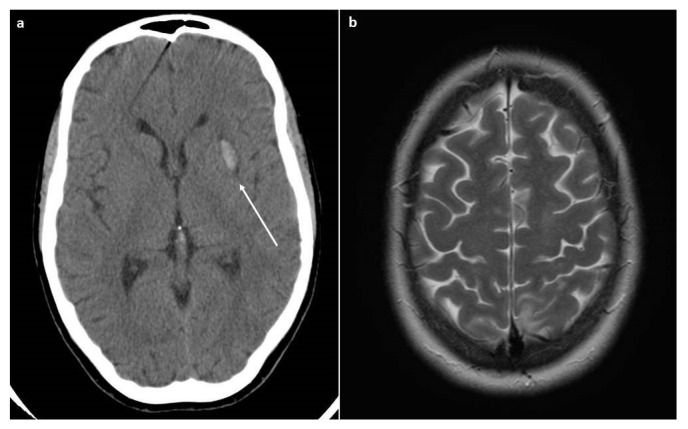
CT and MRI scans. (a) The CT scan revealed the presence of intracerebral hemorrhage (arrow). (b) The MRI scan (Axial T2-weighted image) revealed the presence of cerebral edema that is suggestive of PRES.
